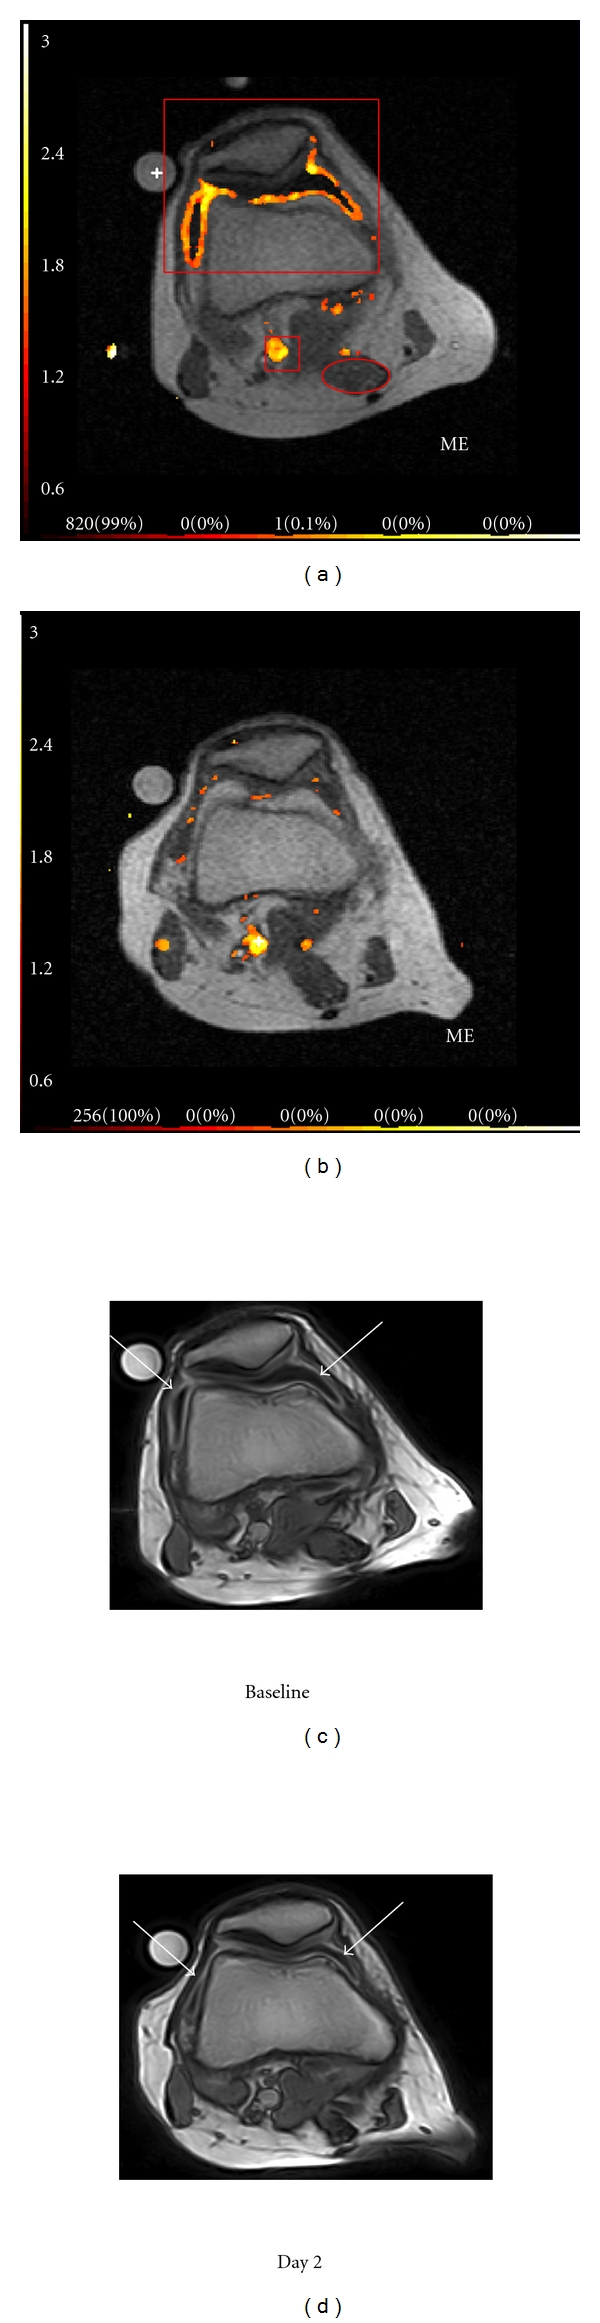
Parametric maps derived from DCE-MRI data maximum enhancement (ME) (a, b) and the corresponding static postcontrast 3D T1-w gradient echo images (c, d) from baseline and day 2. Arrows pointing at the enhancing synovial membrane in the post contrast images (c, d). Examples of the applied ROIs are shown in red in (a) representing the synovial ROI in front of the knee, the popliteal artery ROI behind the knee, and the muscle ROI (oval circle).
